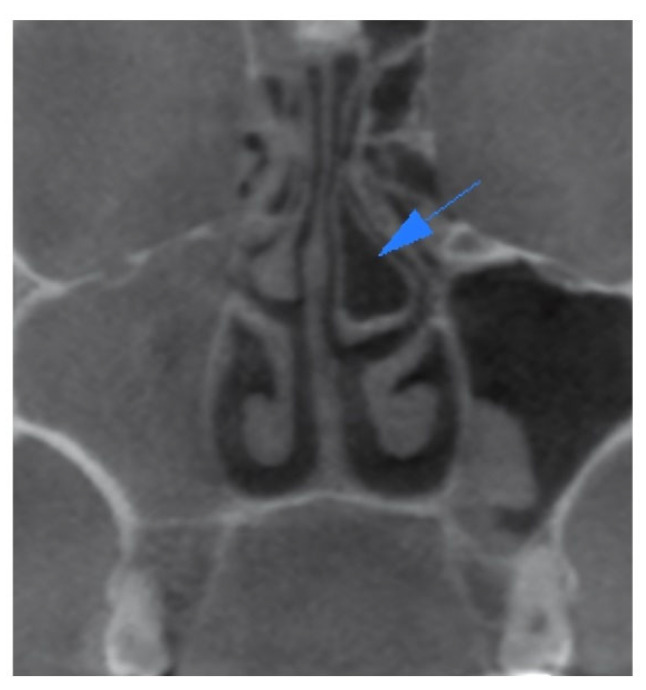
Coronal CBCT scan showing the pneumatization of the middle turbinate, which can be classified as bulbous type of concha bullosa, on the left side marked with blue arrow.
